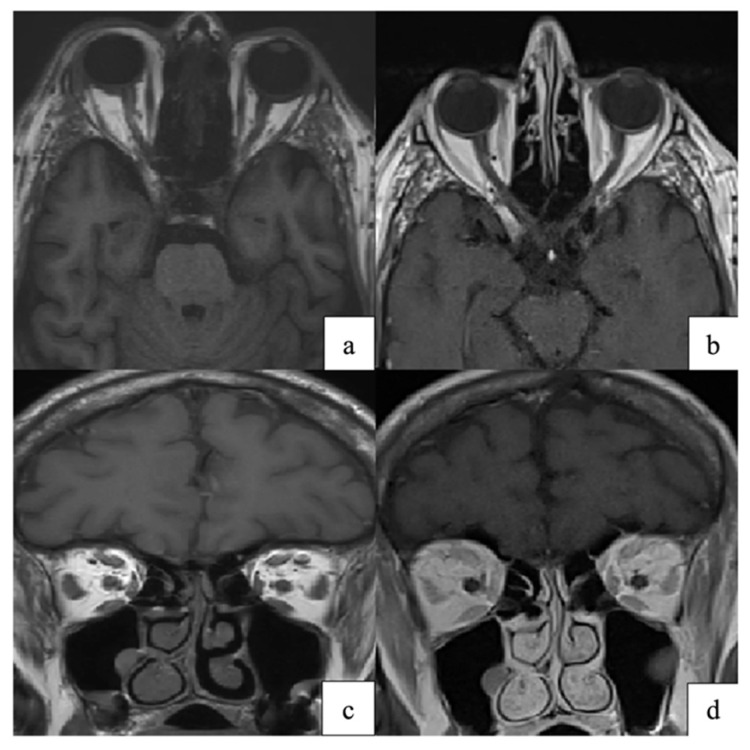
(a,c) Axial and coronal T1-weighted orbital MRI showing altered signal intensity and enlargement of the right superior rectus, medial rectus, lateral rectus, and superior oblique muscles, consistent with orbital myositis following herpes zoster ophthalmicus. (b,d) At one-month follow-up, axial and coronal T1-weighted images demonstrate marked improvement of extraocular muscle myositis. Reproduced from Pace et al., Pathogens 2024, 13, 832, under the CC BY license [79].
